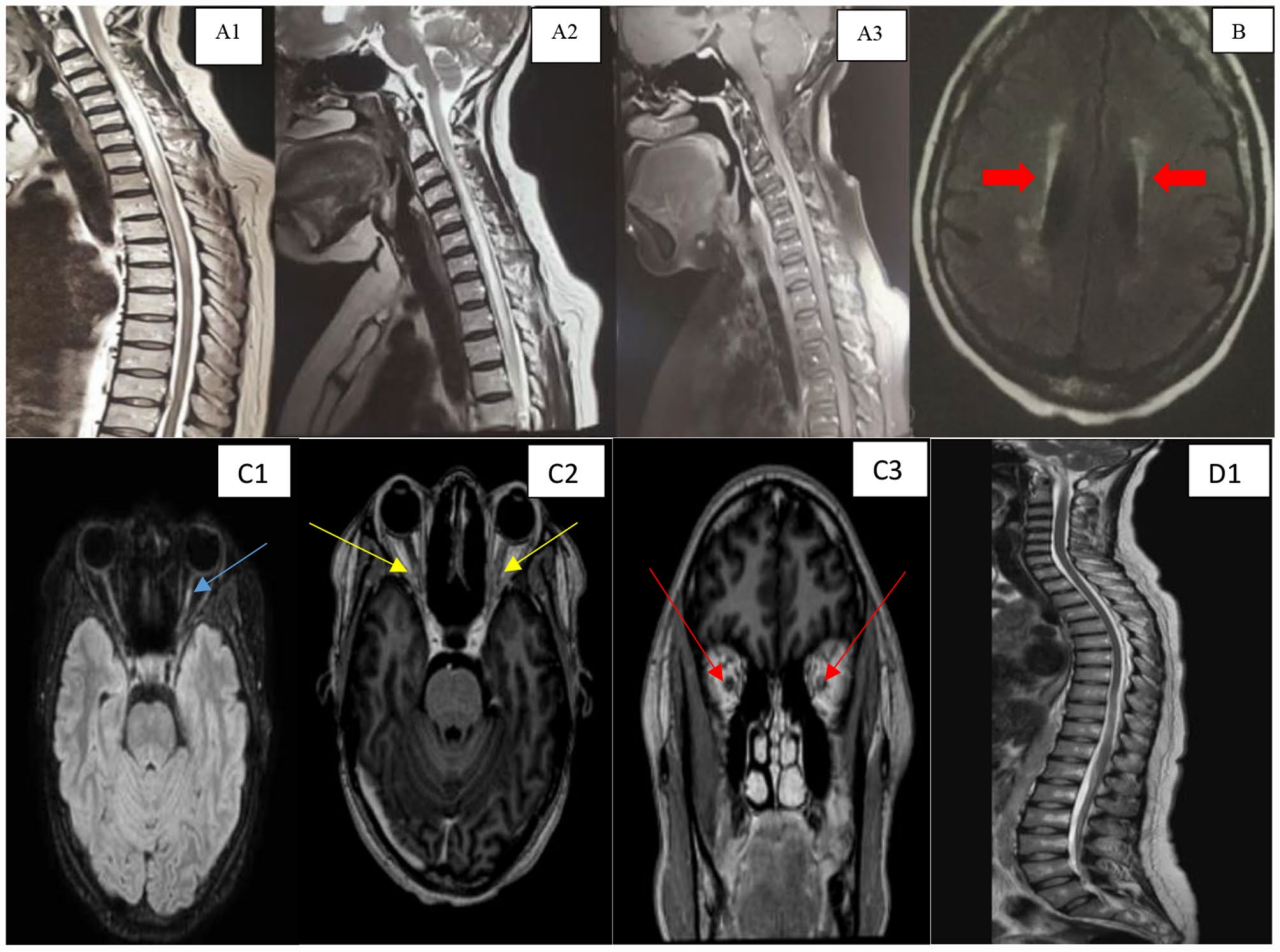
(A1) Sagittal T2-weighted MRI of case one’s cervical and thoracic spine demonstrating longitudinally extensive T2 hyperintensity from C2-T5 with localized spinal cord edema. (A2) Sagittal T2-weighted MRI of case one’s cervical and thoracic spine 8 months later, demonstrating longitudinally extensive T2 hyperintensity from C2-T4 with localized spinal cord edema. (A3) Sagittal T1 gadolinium enhanced MRI of case one’s cervical and thoracic spine at 8 months from initial symptom onset, demonstrating contrast enhancement in the cervical portions of her spinal cord. (B) Axial T2-FLAIR brain MRI of case one, showing periventricular hyperintensities (red arrows) with no significant other parenchymal abnormality. (C1), Axial T2-FLAIR optic nerve and brain MRI of case two demonstrating hyperintensity in the optic nerves, more evident in the left optic nerve (blue arrow). (C2), (C3) Axial and coronal T1-gadolinium enhanced optic nerve and brain MRIs of case two, demonstrating contrast enhancement in both optic nerves, more extensive in her left optic nerve (yellow and red arrows). (D1) Sagittal T2-weighted MRI of case two’s spinal cord, demonstrating normal spinal cord signal
